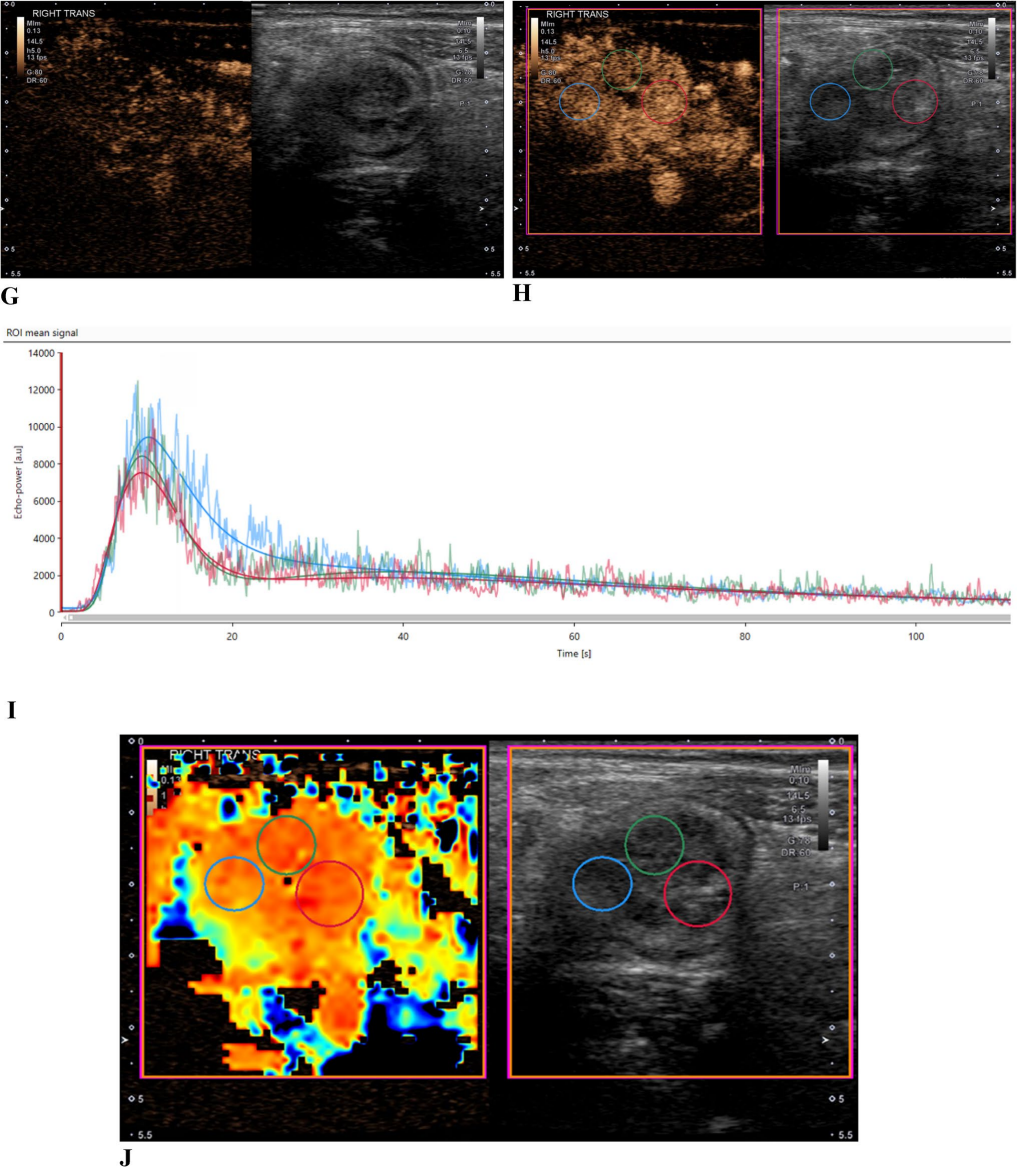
A 19-year-old with ileal Crohn’s disease. a Axial single-shot fast spin-echo MR image shows terminal ileal wall thickening and intramural edema (arrow). b Axial postcontrast T1-weighted image shows marked hyperenhancement (arrow). c-g Side-by-side contrast-specific and gray-scale contrast-enhanced ultrasound images at 0 s, 15 s, 25 s, 60 s, and 120 s show wash-in and wash-out of contrast material in the bowel wall over time. h Ultrasound image at peak enhancement shows three round regions of interest in the bowel wall. i Time vs. signal intensity curves from the three regions of interest show bowel wall wash-in and wash-out of microbubble contrast agent. j Contrast-enhanced ultrasound peak enhancement parametric map shows relative enhancement of the bowel wall compared to adjacent structures
